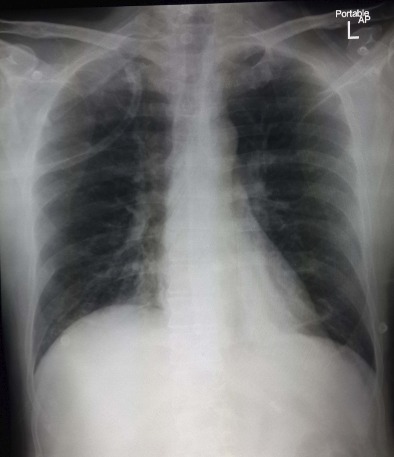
Chest X-ray revealed aberrations, showing bilateral discrete shadowing, similar to atypical pneumonia
